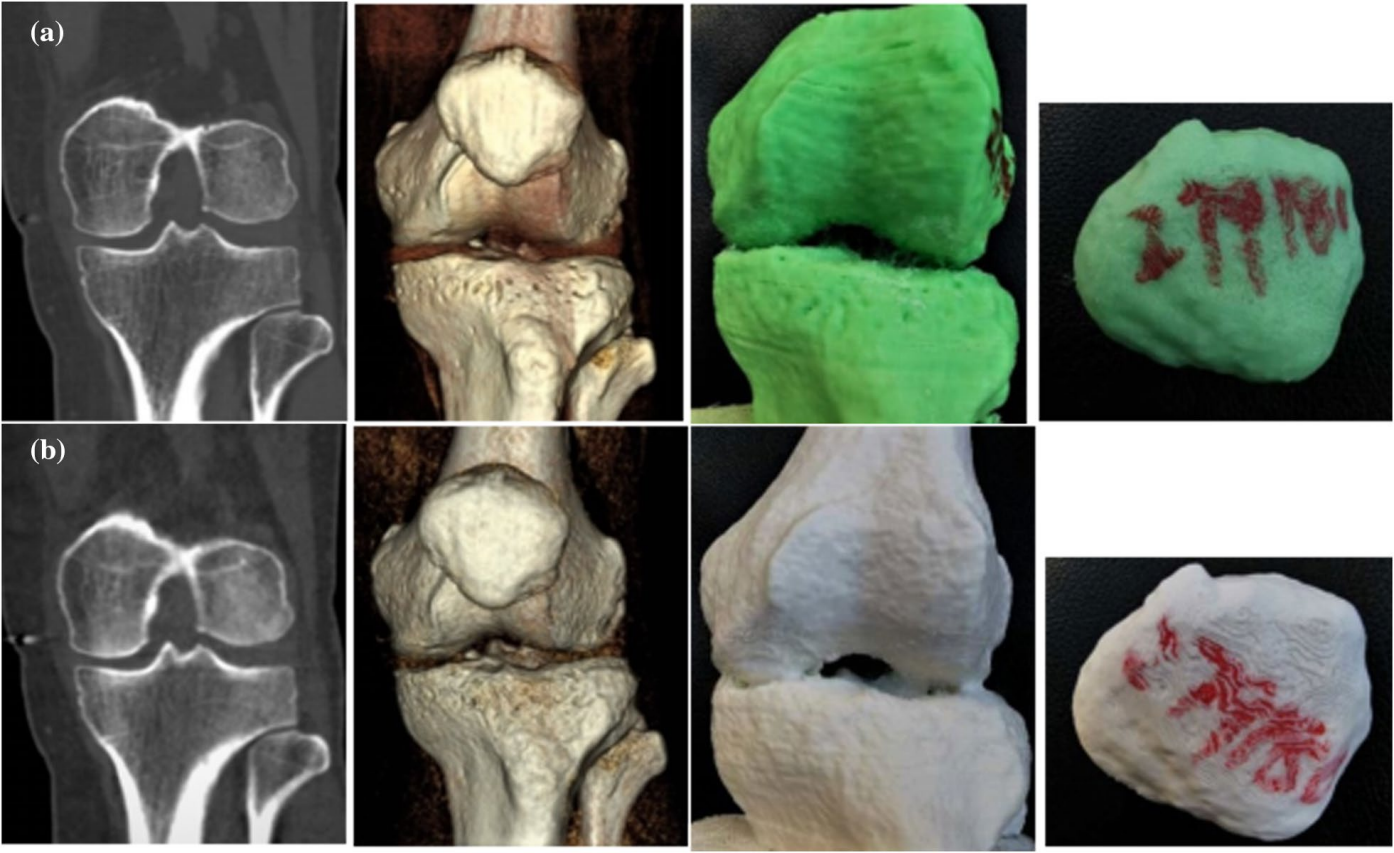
Representative case study 1. A 62-year-old man with a suspected intercondylar ridge fracture underwent two scans (at the first visit and 7 days later). Standard-dose (a) and ultra-low-dose (b) protocols were used to generate three-dimensional printing models based on 3D CT data. Image quality and 3D printing model based on the former is better than the latter (3 vs. 2 points). The 3DP model is appropriate for preoperative planning
